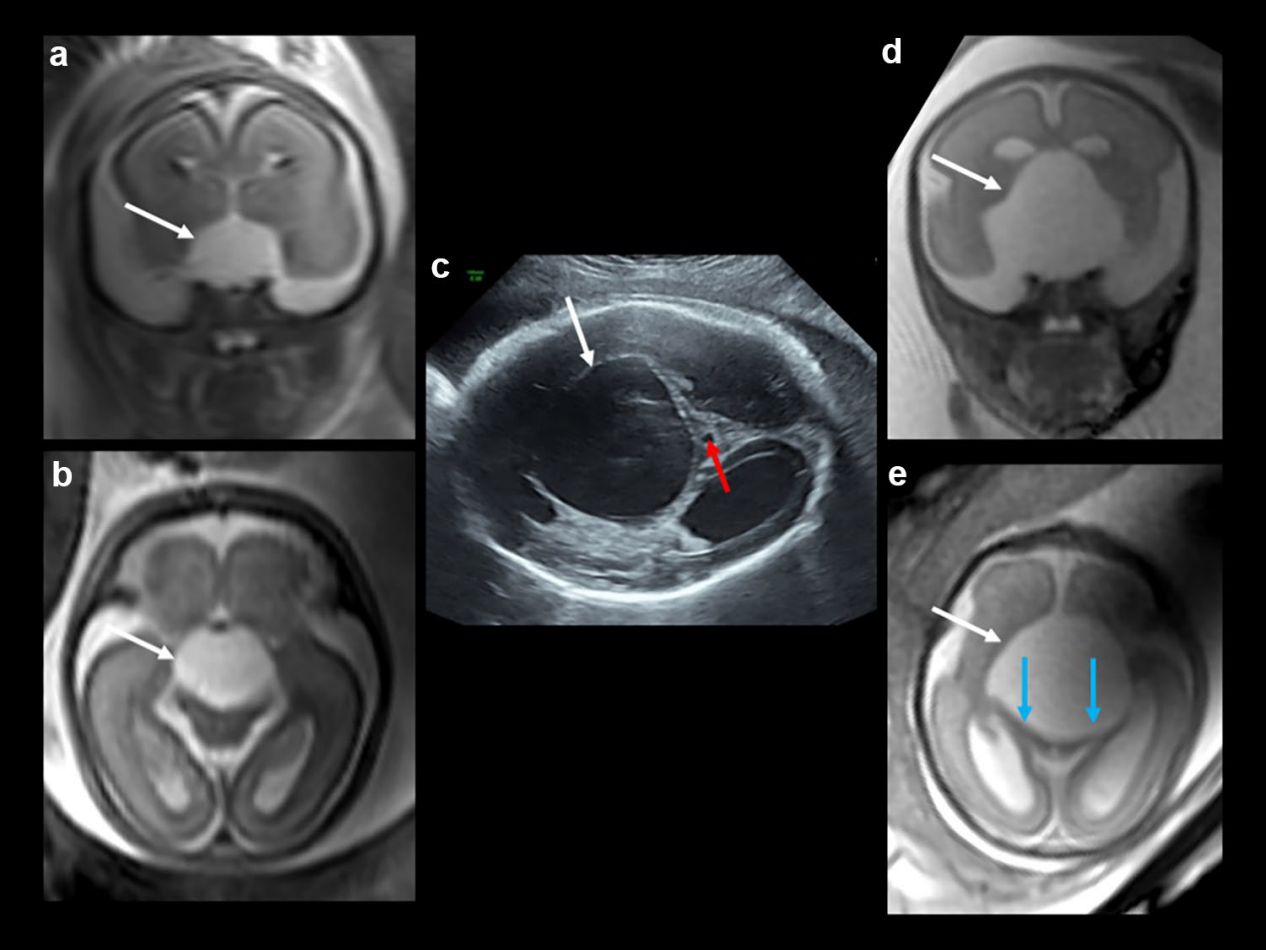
a, b Prenatal MRI at 21 weeks of gestational age (T2-weighted) in coronal (a) and in axial (b) orientations (courtesy of PD Dr. K. Kreiser, Technical University, Munich): suprasellar midline cystic formation (white arrows) with moderate displacing character. c Prenatal ultrasound at 31 + 1 weeks of gestational age: suprasellar arachnoid cyst (white arrow) of up to 5-cm diameter with displacement of the surrounding brain structures and occlusion of the aqueduct (red arrow) with subsequent hydrocephalus. d, e Prenatal MRI at 26 weeks of gestational age (T2-weighted) in coronal (d) and in axial (e) orientations (courtesy of PD Dr. K. Kreiser, Technical University, Munich): significant increase in size of the suprasellar cystic formation (white arrows) now with clear displacement of the cerebral peduncles (e, blue arrows)
